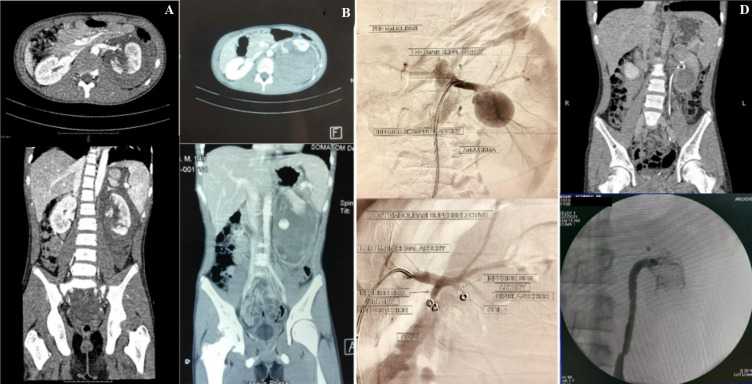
evaluation and clinical findings in the third patient A) initial CT scan showed laceration of left kidney with a form of shattered kidney: coronal view (upper), axial view (lower); B) 1-week urgent CT scan evaluation left kidney hematoma with a decrease of renal function secretion was found without any active bleeding and leakage: coronal view (upper), axial view (lower); C) super selective embolization; D) abdomen CT scan evaluation 1 month after drainage of hematoma and embolization (upper), RPG evaluation during DJ stent removal (Lower)
